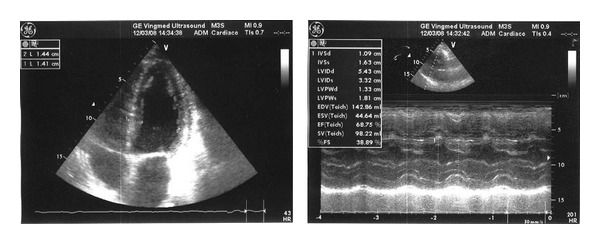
Case 2: echocardiography after the bortezomib cycles.
